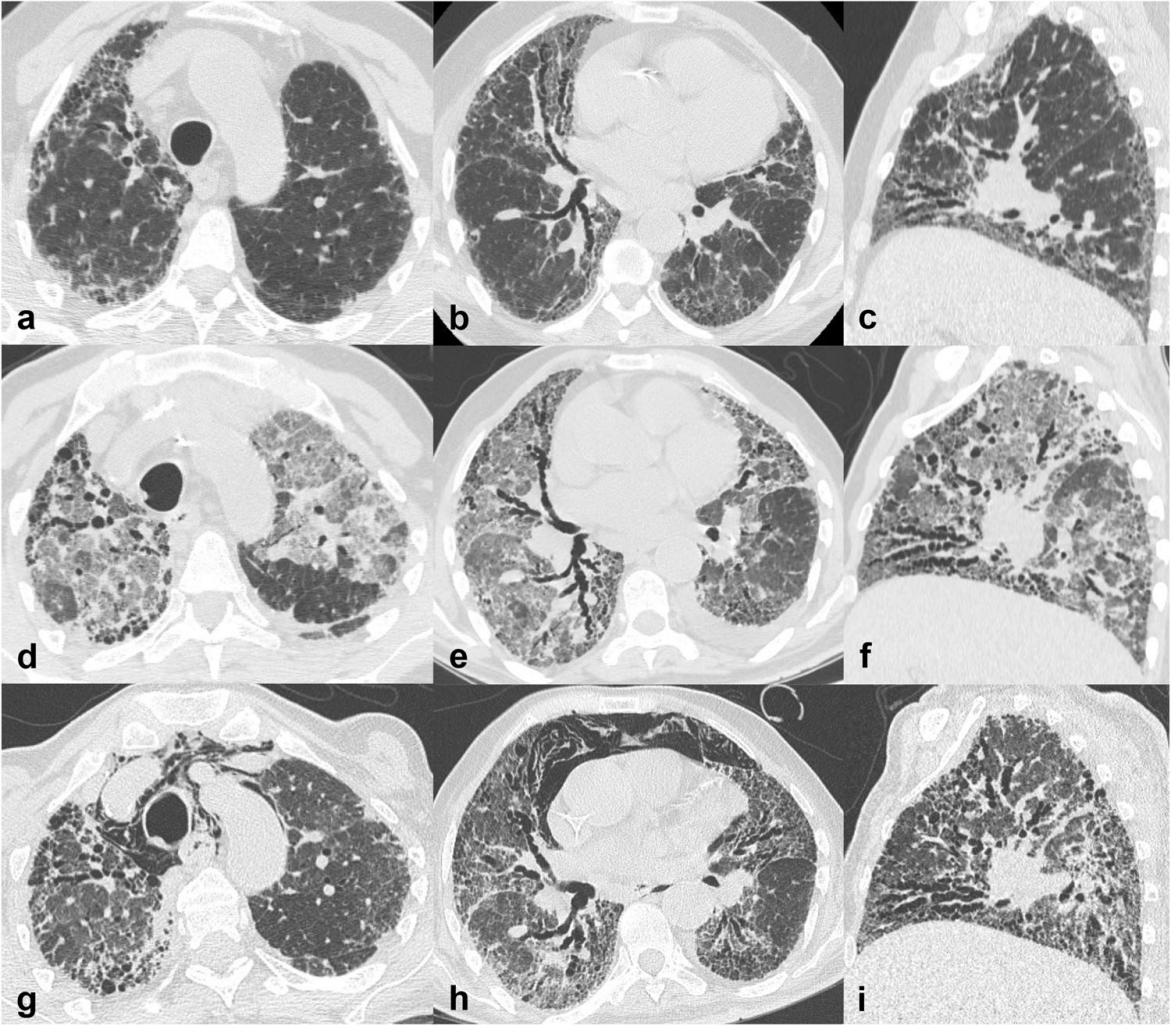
Acute exacerbation of idiopathic pulmonary fibrosis (IPF). Axial and sagittal computed tomography (CT) images (a–c) depicting a usual interstitial pneumonia (UIP) pattern with the typical peripheral predominant distribution, in the mid-lower posterior zones and antero-superior zones of the lungs (c). Honeycombing is evident in the anterior segment of the right upper lobe (a). Axial and sagittal CT images (d–f) performed 15 months later in the emergency department for acute respiratory distress of the patient show the appearance of almost diffuse areas of ground-glass opacity in both lungs, mostly in the areas relatively spared by fibrosis, suggestive of acute exacerbation. After 40 days from the acute phase, a new chest CT scan was requested for persisting chest pain. Axial and sagittal CT images (g–i), analysed at the same anatomic levels as before, demonstrate the presence of spontaneous pneumomediastinum, explained by the alveoli rupture and interstitial emphysema due to distress; interesting to note the evident progression of pulmonary fibrosis respect to the previous exam, which is a common occurrence after an acute exacerbation
